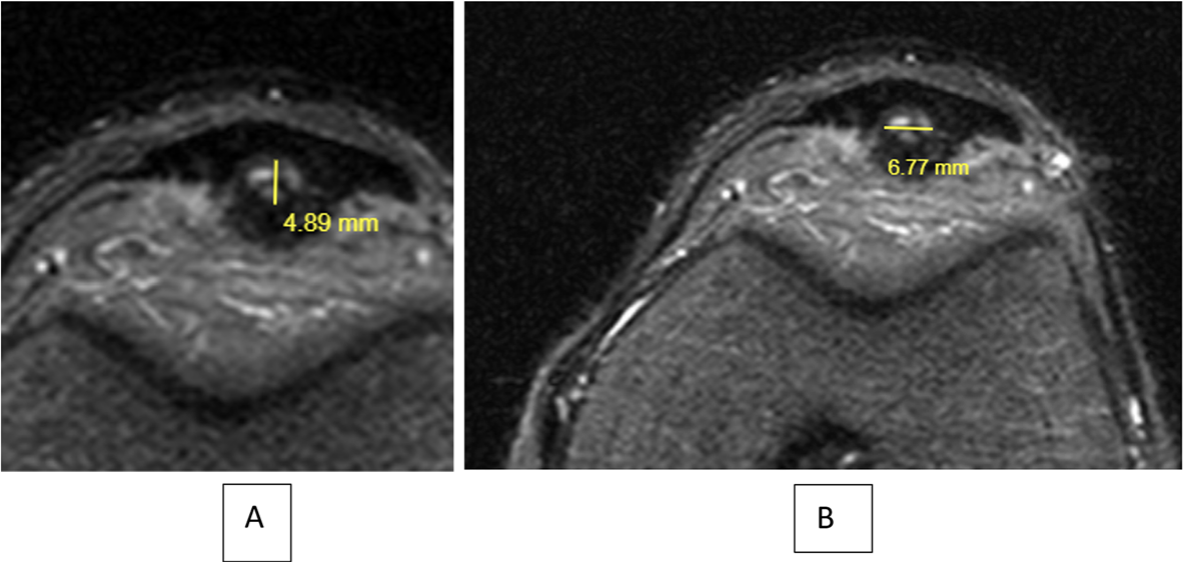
A MRI evaluation: Thickness of the tear. B Width of the tear
